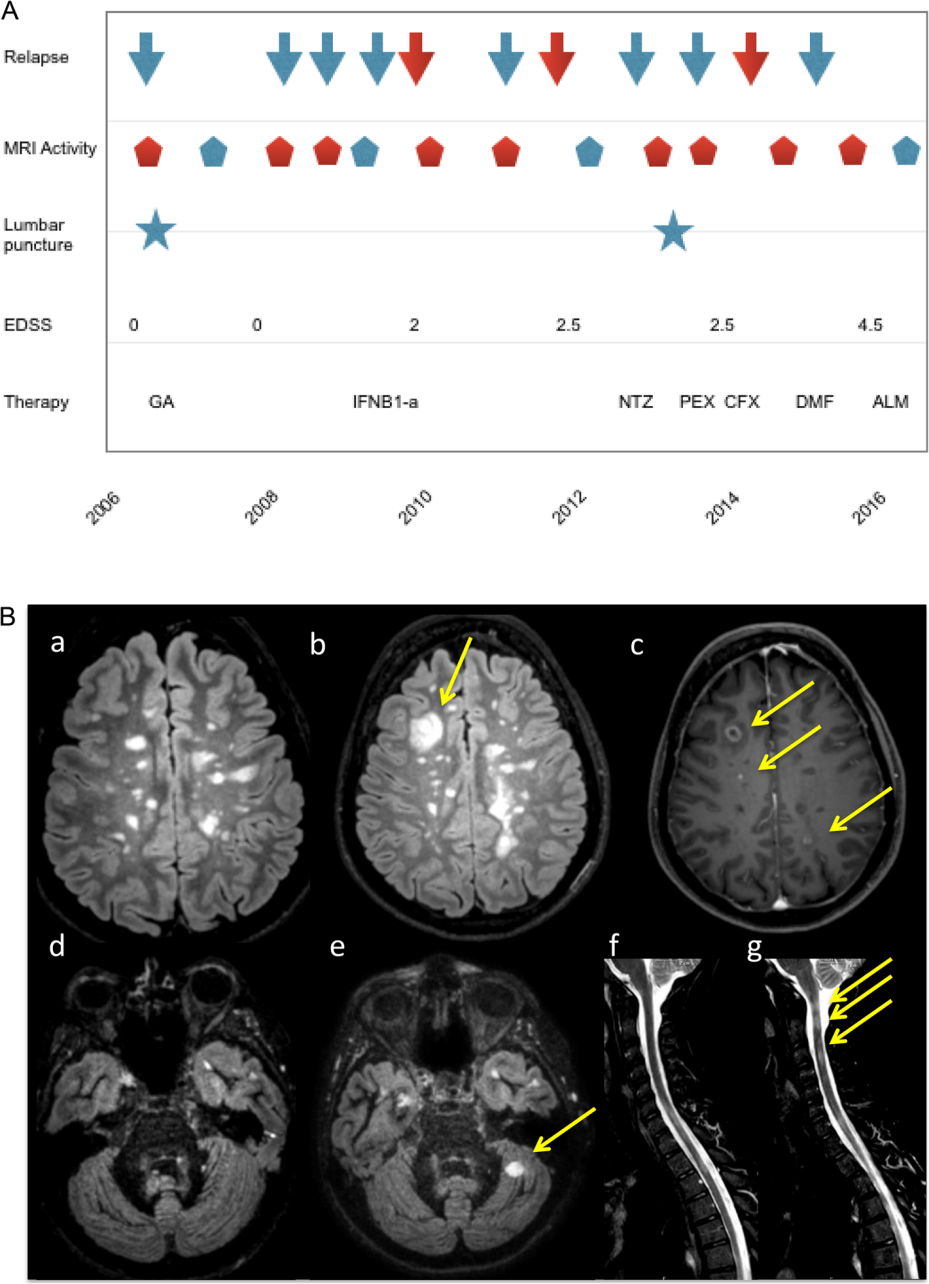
(A) Patient’s history was characterized by sustained disease activity. Red arrows correspond to relapses, which caused disability accumulation. Red pentagons correspond to disease activity at MRI follow up characterized by new T2 lesions or T1 Gd-enhancing lesions. Lumbar puncture was performed in 2006 and 2013. GA Glatiramer acetate; IFNB1a Interferon Beta-1a; NTZ Natalizumab; PEX Plasma Exchange; CFX cyclophosphamide; DMF Dimethyl-fumarate; ALM Alemtuzumab. (B) Brain 3 T-MRI obtained at the time of second lumbar puncture (a, d, f) and during a subsequent follow up of 2015 (b, c, e, g). Axial 3D Fluid Attenuated Inversion Recovery (FLAIR, a, b), post contrast T1w (c), 3D Double Inversion Recovery (d, e), spinal cord STIR (f, g) are shown. Arrows indicate new lesions
